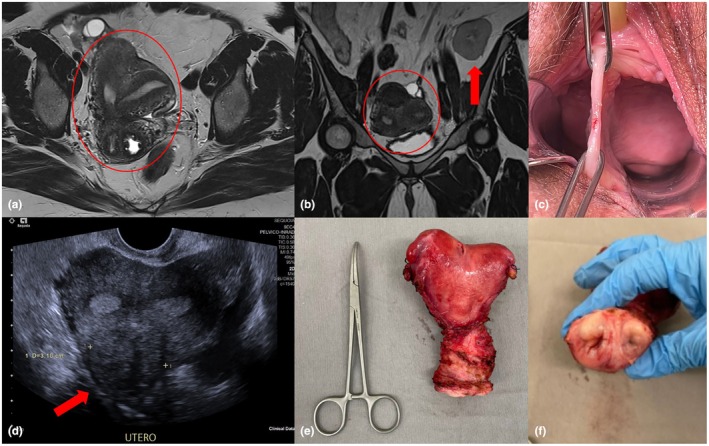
(a) Didelphic uterus (red circle) in an axial cut of MRI. (b) Didelphic uterus (red circle) and transplanted left kidney (red arrow) in a coronal cut of MRI. (c) Non‐obstructive longitudinal vaginal septum. (d) Didelphic uterus and fibroids (red arrow) in an axial cut of a transvaginal ultrasound. (e) Anatomic view of didelphic uterus. (f) Inferior view of didelphic uterus. MRI, magnetic resonance imaging.
